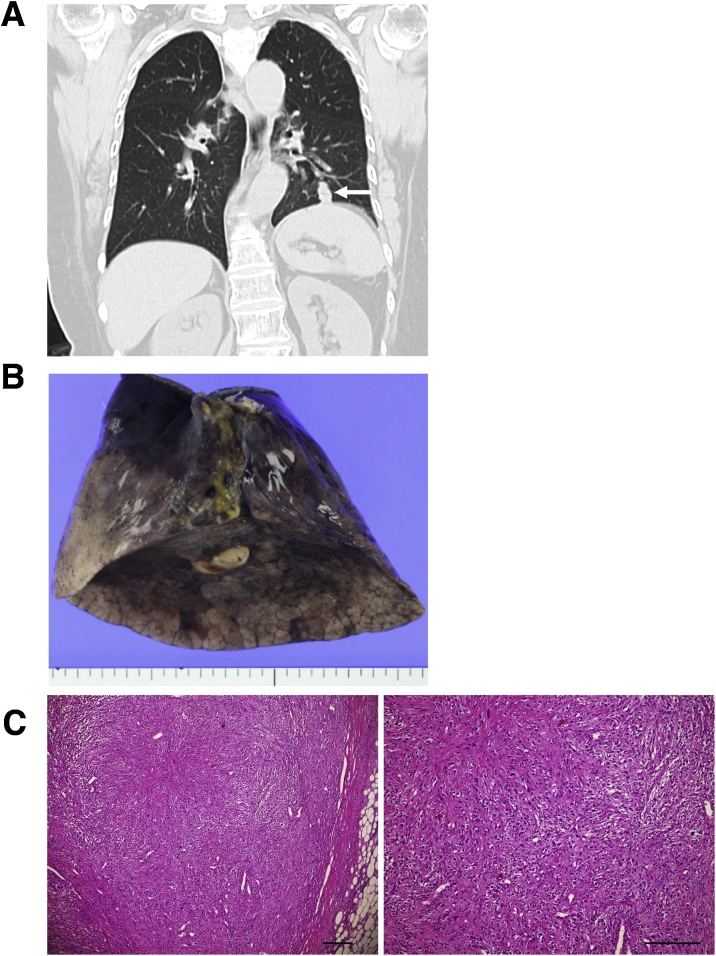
A. A HRCT of the chest detected a nodule with a maximum diameter of 22 mm in the left lower lobe of the lung. B. Macroscopic findings of the metastatic tumor. The nodule appears white and lobular. Pathologically, no pleural invasion was observed. C. Pathological diagnosis of the tumor was metastasis from DDLS. Scale bar = 200 μm.
